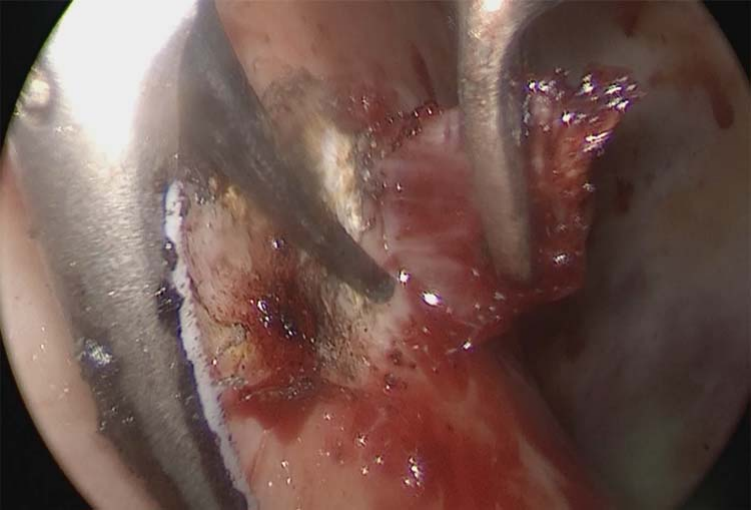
Intraoperative thoracoscopic image, and the diaphragm layer was peeled off with the active blade of the ultrasonic coagulation and incision device.
